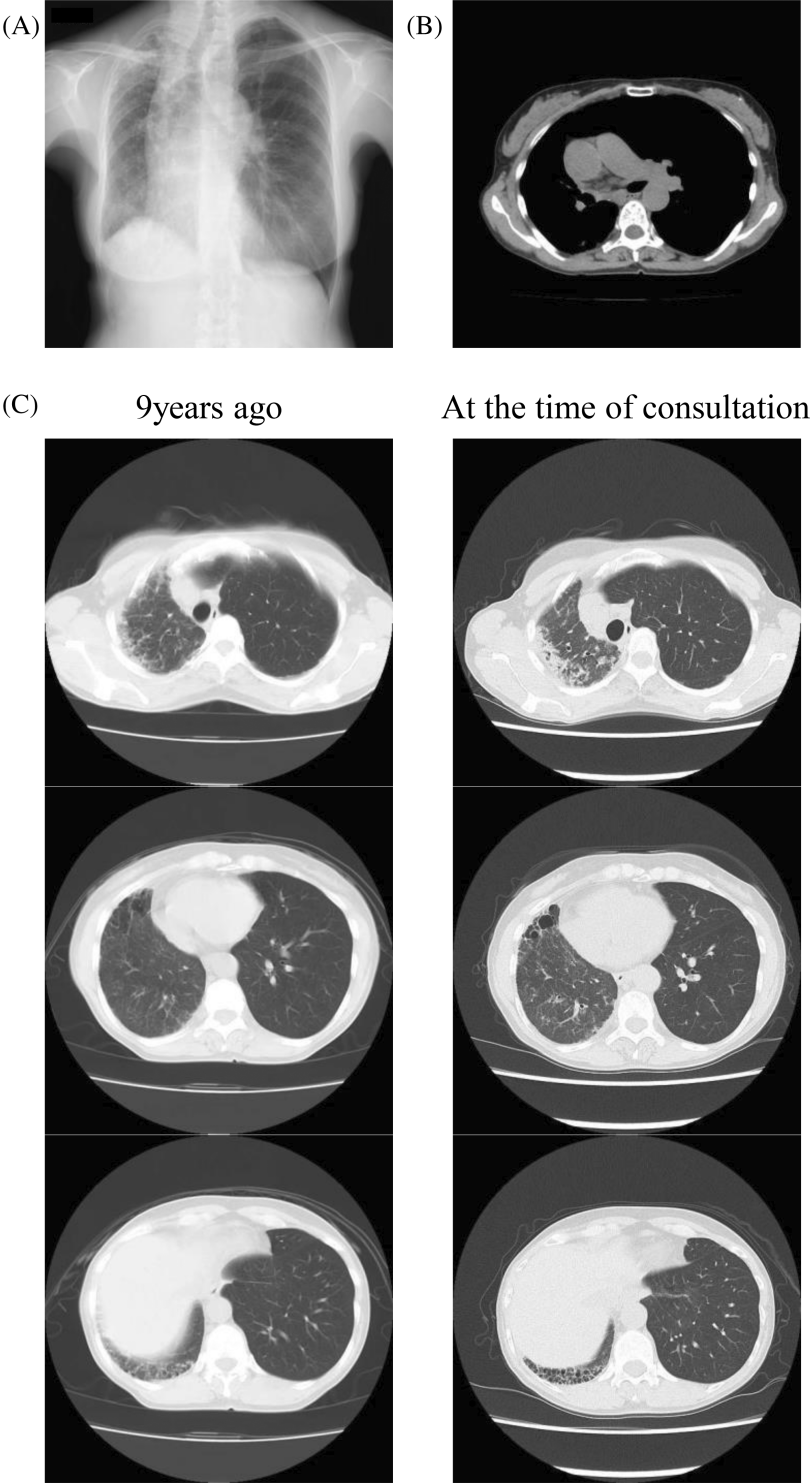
(A) Asymmetry of the thoracic cage with mediastinal shift to the right, ground‐glass opacity, and infiltrative shadow in the upper right lung field, along with reticular shadowing in the right lower lung field. (B) The right pulmonary artery is not visible. (C) Time series of chest computed tomography images: Left column shows images from 9 years ago; right column shows images from the time of consultation. Consolidation in the right upper lobe, cystic changes in the right middle lobe, and honeycombing in the right lower lobe have progressed over time.
